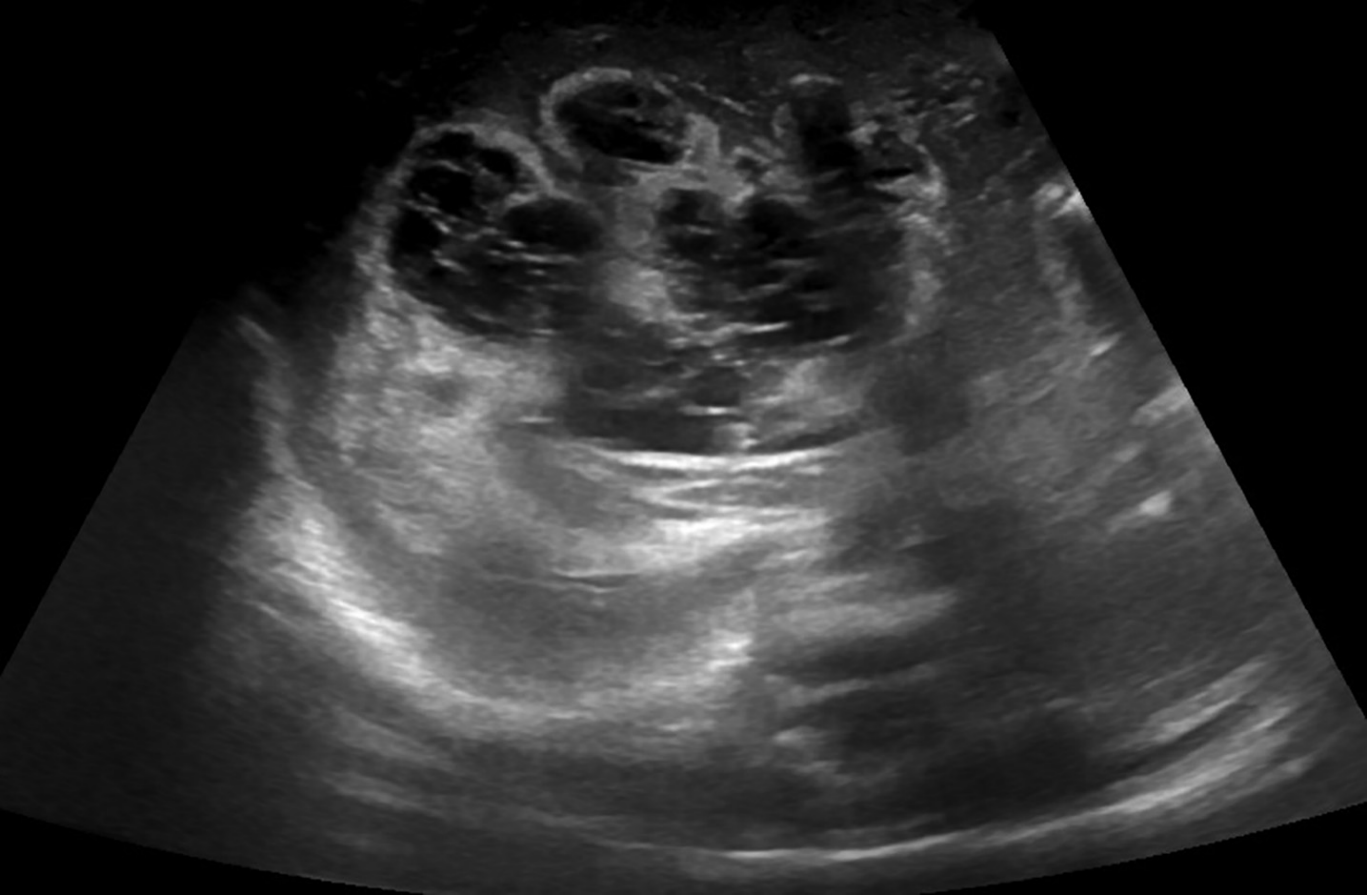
Ultrasound image with the multicystic lesion of the liver's right lobe.
